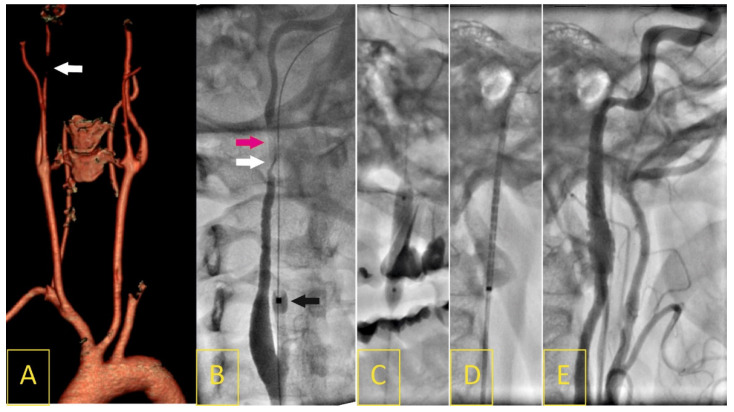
A 45-year-old woman who had been unsuccessfully treated for several years for headaches and “swishing” noises in her right ear presented with a 12-day history of right-hemisphere ischemic stroke (4 points in the National Institute of Health Stroke Scale). A Doppler ultrasound image on admission revealed long, irregular, string-sign right internal carotid artery (RICA) stenosis in its intracranial segment, with a peak systolic velocity of 5.28 m/s and end-diastolic velocity of 2.53 m/s, corresponding to lumen stenosis of more than 90%. Computed tomography angiography confirmed a 5 cm long 95% RICA stenosis with “string-of-beads” morphology typical for FMD (A). Catheter angiography showed RICA string-sing stenosis (white arrow) with local dissection (pink arrow, (B)); black arrow indicates distal balloon of proximal neuroprotection system (9F Mo.Ma). Under flow reversal, the stenosis/dissection segment was crossed with a 0.014” wire, then predilated with a 4 × 20 mm semi-compliant balloon (C). A self-expanding 8 × 30 mm stent (Precise) was deployed (D). Angiography (E) demonstrated effective reconstruction of the RICA lumen with significant improvement to the distal flow (TICI-3).
